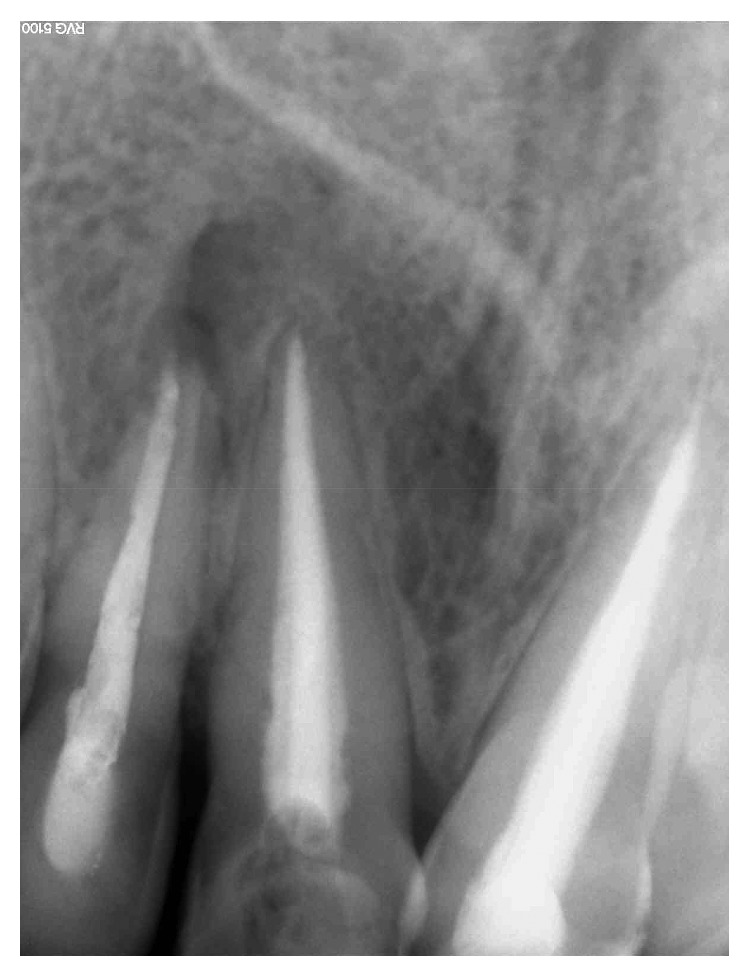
Radiograph of control 3 years later.
